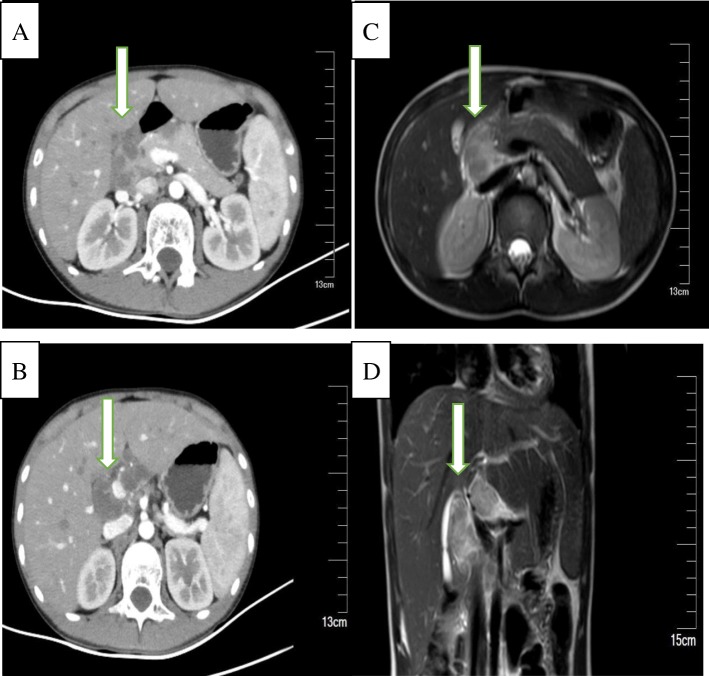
Both abdominal enhanced CT (a, b) and MRI imaging (c- axial, d- coronal) demonstrated occupied lesions in the hepatic hilum (3.0*2.7 cm), some of which are mixed, and the un-enhancing, central, low density lesion (from the hepatic hilum to the middle of the common bile duct, surrounding the hepatoduodenal ligament)with the appearance of an enhancing peripheral rim
